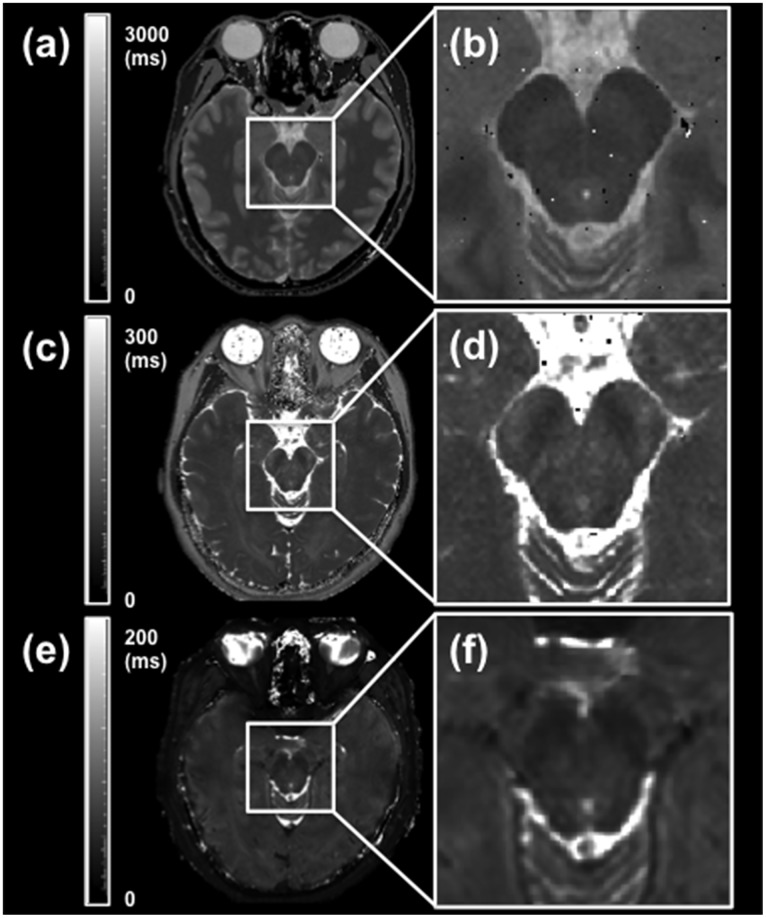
Typical quantitative T_1_, T_2_, and T_2_* maps of the lower midbrain. (a) Typical T_1_ map. The gray scale represents the T_1_ values from 0 to 3000 ms. (b) Enlarged T_1_ map. (c) Typical T_2_ map. The gray scale represents the T_2_ values from 0 to 300 ms. (d) Enlarged T_2_ map. (e) Typical T_2_* map. The gray scale represents the T_2_* values from 0 to 200 ms. (f) Enlarged T_2_* map. The location of the single slices of the T_1_, T_2_, and T_2_* maps was the same as the location of the neuromelanin-sensitive MRI slice.
